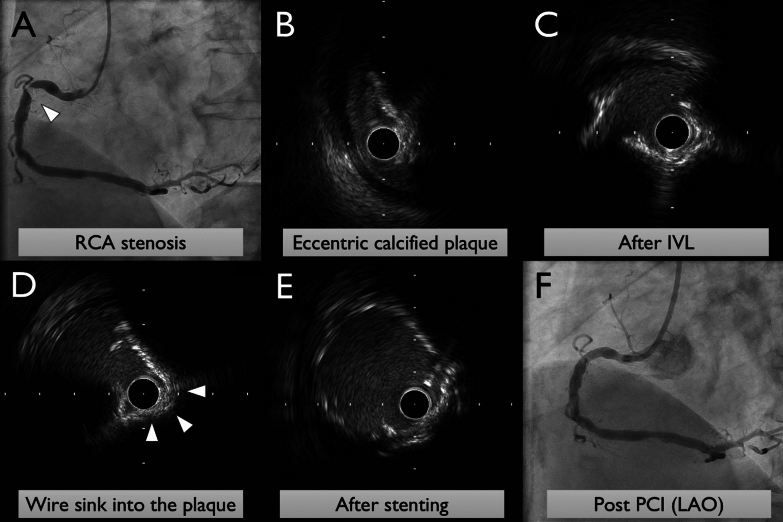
Images of coronary angiography and intravascular ultrasound during primary percutaneous coronary intervention. A Coronary angiography (CAG) showing severe, calcified, eccentric stenosis in the right coronary artery. B Intravascular ultrasound (IVUS) image reveals the eccentric calcified lesion. C The guidewire and IVUS catheter are partially embedded within the concavity of the calcified nodule. D IVUS image after expansion using a 4 mm balloon, showing expanded calcified crackles and the wire sinking into the calcified plaque. E Successful expansion and placement of a 5 mm × 15 mm drug-eluting stent. F Final CAG demonstrated good stent expansion
